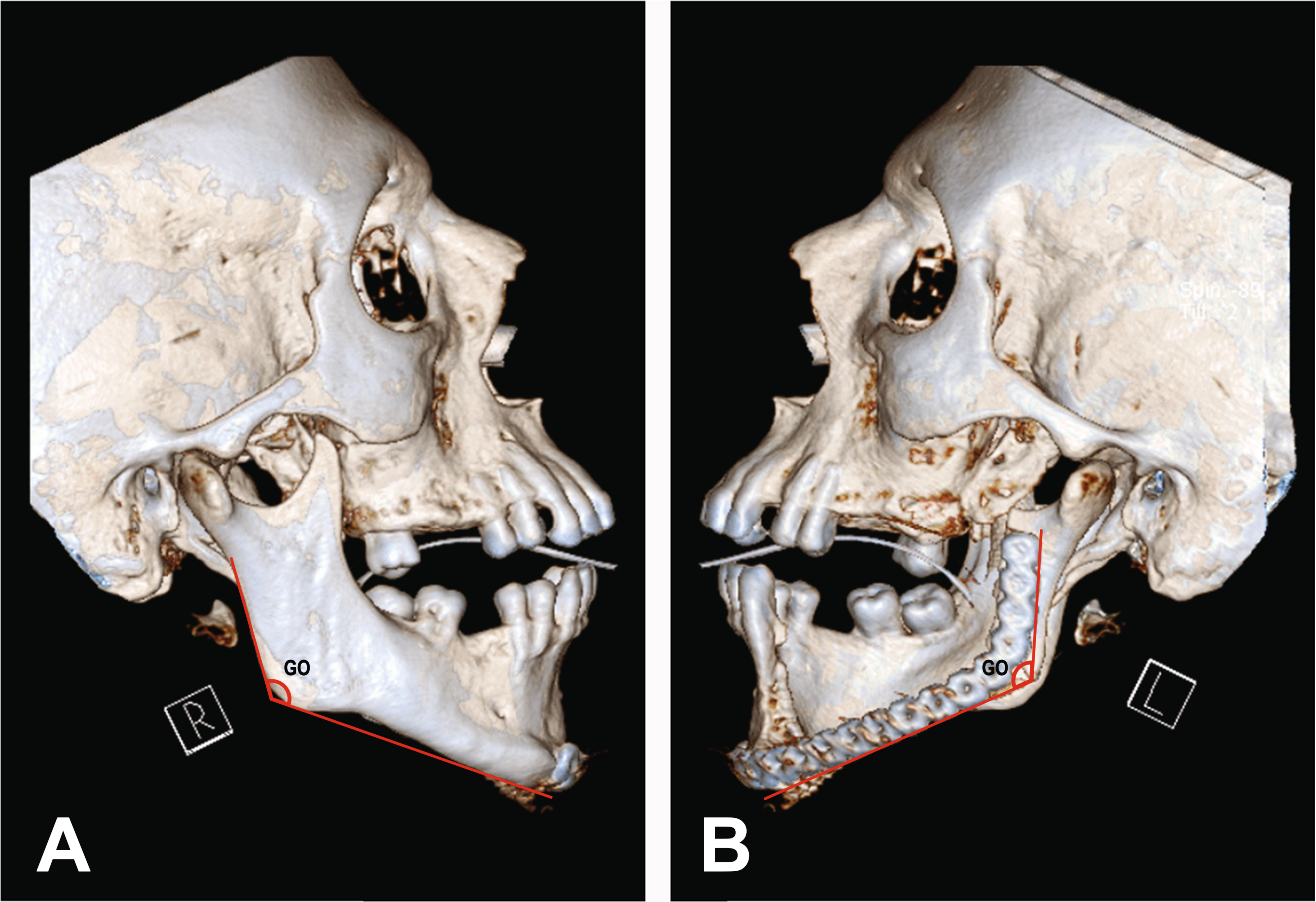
a The measurement of a non-affected gonial angle in 3D-CT images (sagittal view), and b for reconstructed gonial angle
